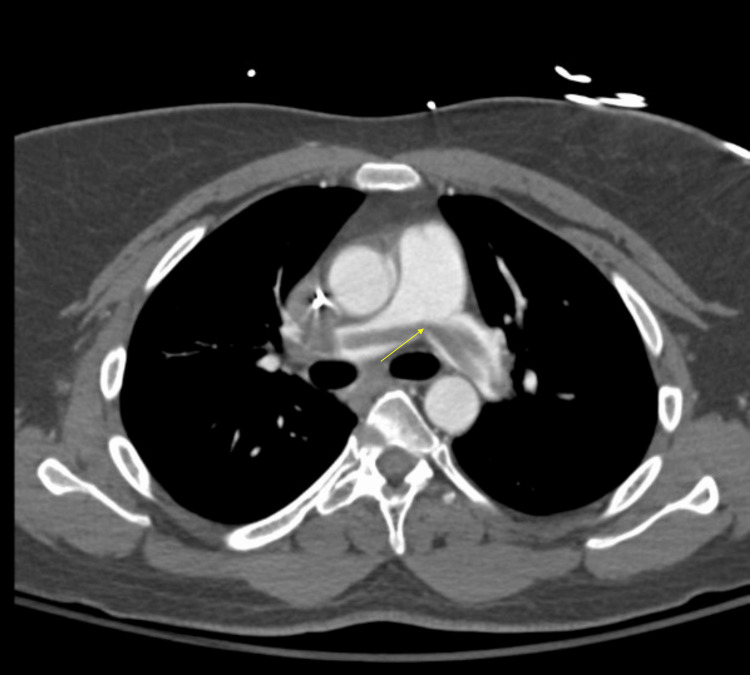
CT angiography chest on hospital day four demonstrated a large saddle pulmonary embolus (yellow arrow) extending from the main pulmonary artery to the bilateral segmental pulmonary arteries.
